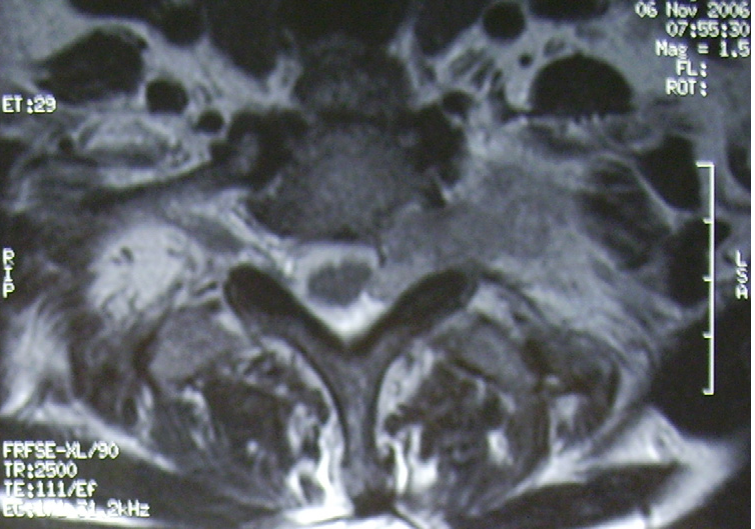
Transverse section of T1 weighted MRI demonstrating protrusion of the tumor from the left T1-2 intervertebral foramen to the spinal cord.
